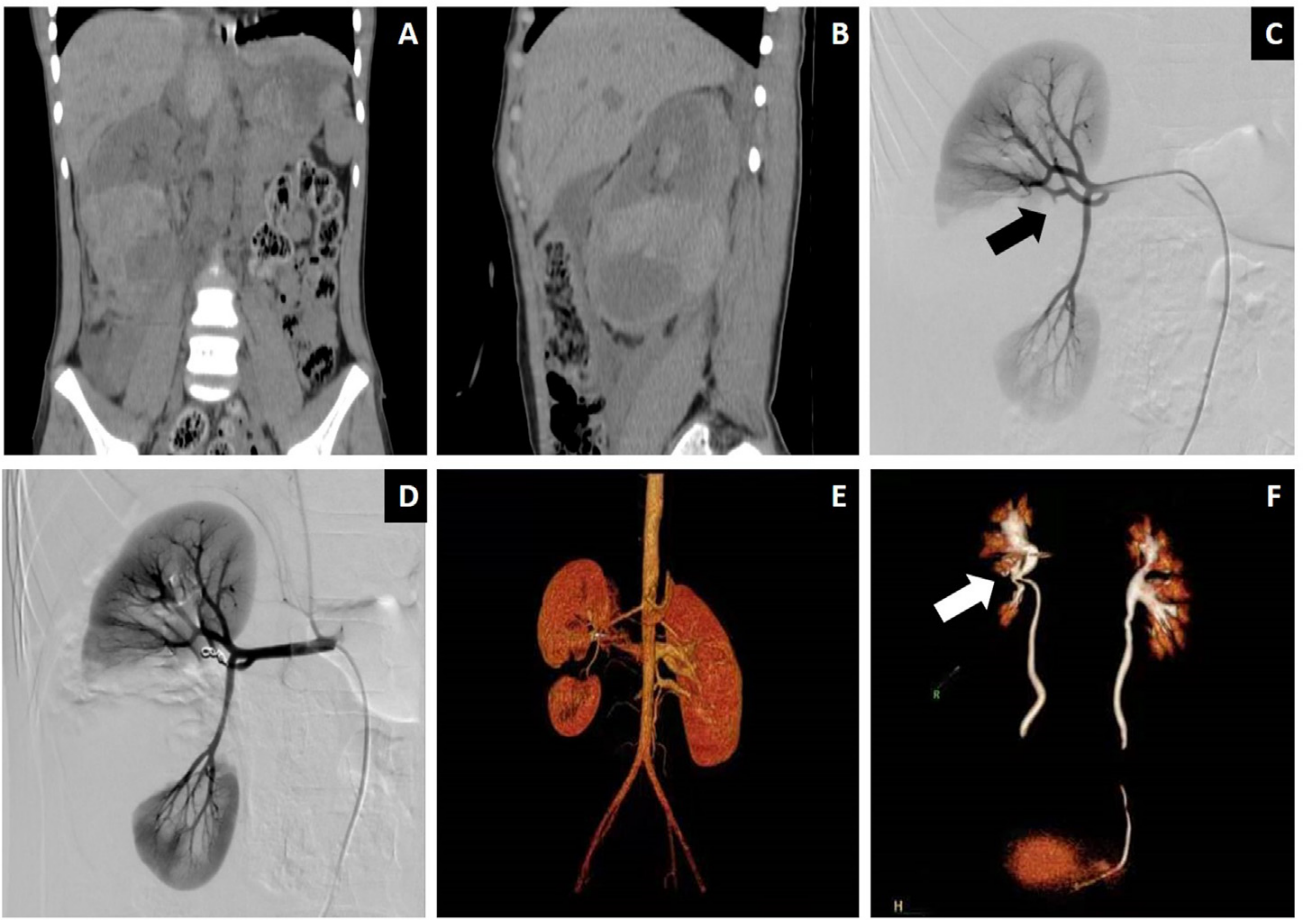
Images of a representative case with renal injury (AAST Grades V). (a, b) Primary CT scan revealed a completely shattered right kidney. (c) DSA showed vessel disruption located at the segmental arteries (black arrow). (d) The involved arteries were embolized by GS plus coils. (e, f) Follow up contrast-enhanced CT along with volume reconstruction showed a completely shattered right kidney and renal pelvis stenosis (white arrow).
